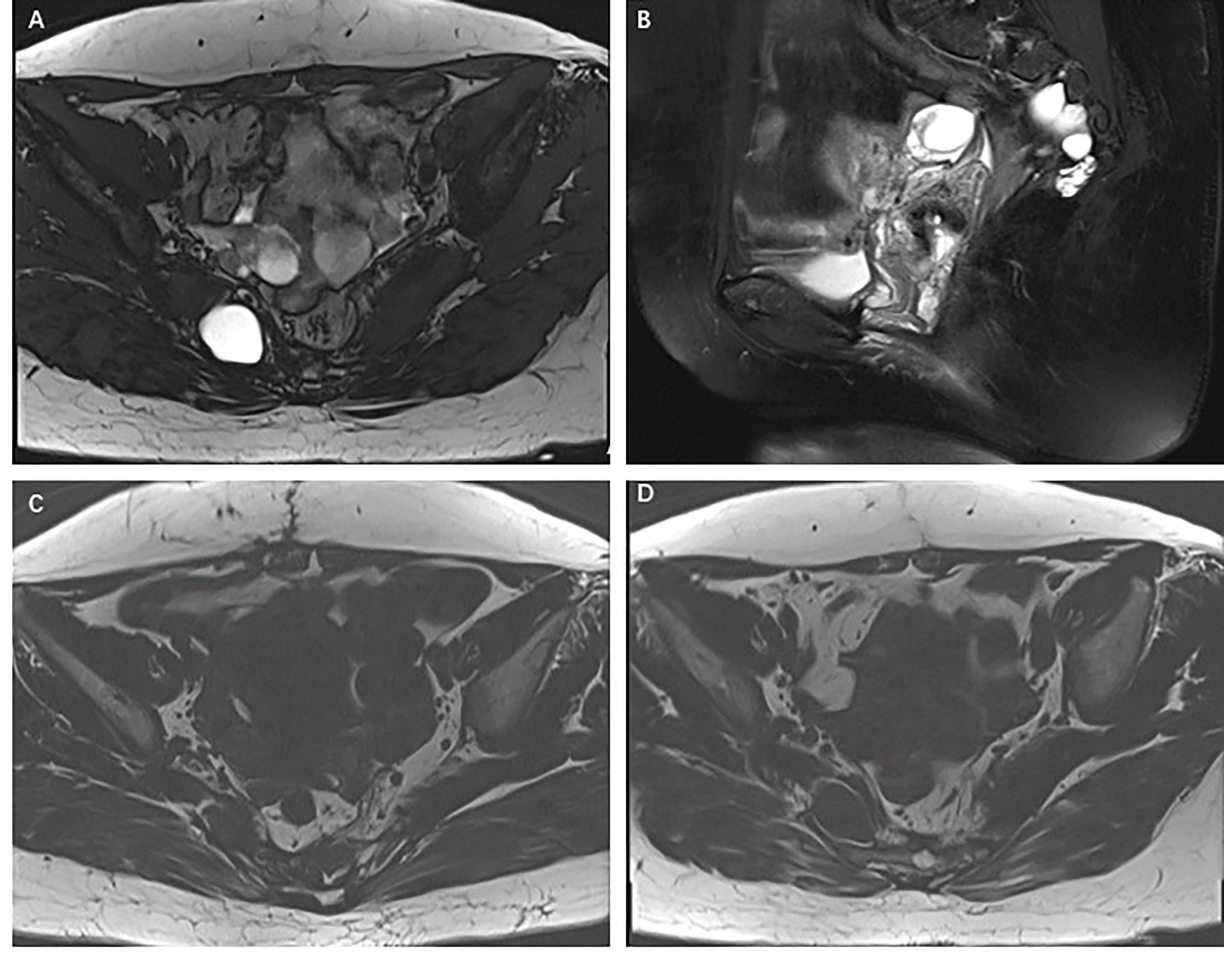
(A–C) T1- and T2-weighted MRI scans demonstrating multiple ventricular T2 hypersignal and T1 hypointense cystic lesions in the right buttock tissue and sacrococcygeal region. (D) T2-weighted gadolinium-enhanced MRI scans demonstrating annular enhancement of the cystic wall, but no enhancement of the cystic part of the lesion.
